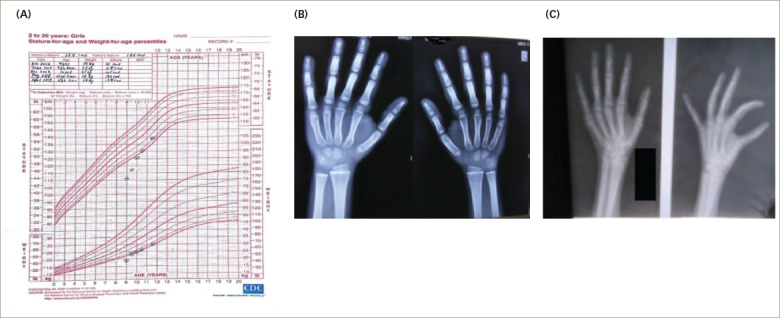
Basic endocrine work-up Case 2. A: Growth chart; B: X-ray at diagnosis; C: X-ray at 12 months post-treatment
